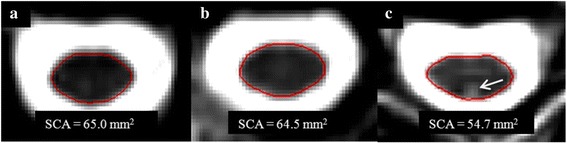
Sample images and segmentations results. Representative baseline cervical spinal cord T2-weighted axial images (taken at C3) from three patients with RRMS on interferon β-1a treatment. The corresponding region-of-interest (*red oval*) resulting from the semiautomated cord contouring tool is also shown. The normalized spinal cord area (SCA) for each patient, determined from C1-C5, is listed below the images. a A patient with a disease duration (DD) of 19.4 years, Expanded Disability Status Scale (EDSS) score of 2.0, and timed 25-foot walk (T25FW) of 4.0 s. b A patient with DD 4.4 years, EDSS 2.5, and T25FW 5.7 s. c A patient with DD 24.4 years, EDSS 1.5, T25FW 4.0 s, in whom successful spinal cord contouring was performed despite the presence of a T2 hyperintense spinal cord lesion (indicated with *arrow*)
